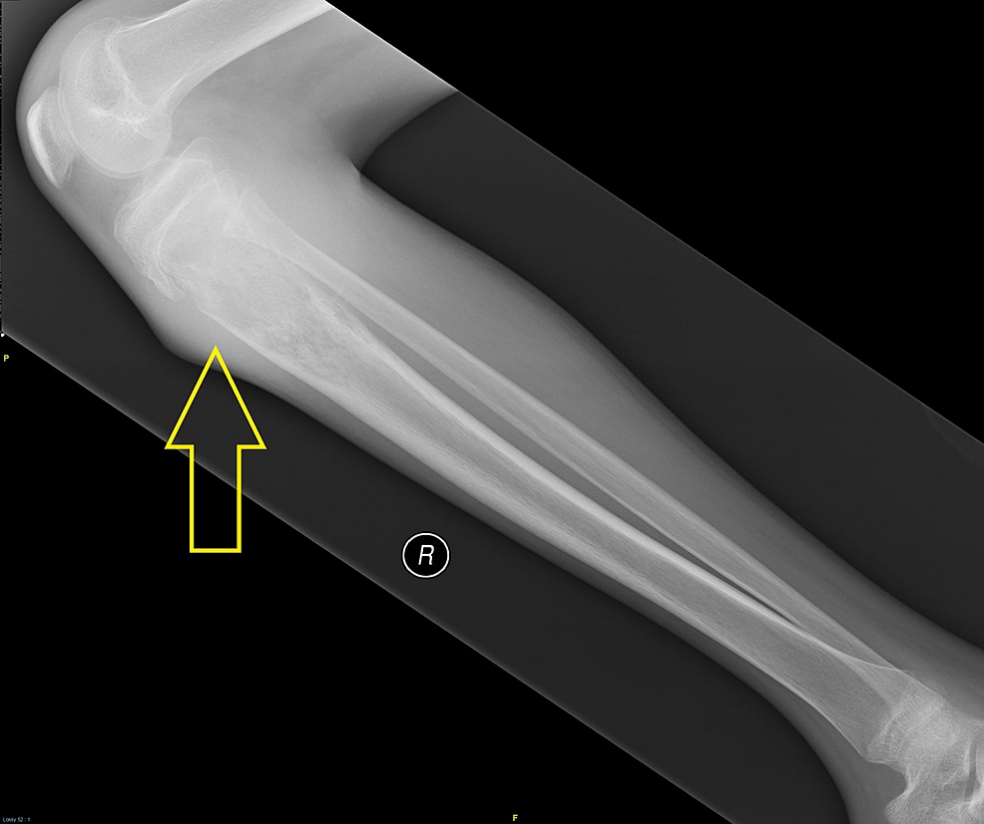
X-ray shows an ill-defined lytic lesion at the proximal third of the right tibia with periosteum elevation (yellow arrow).
